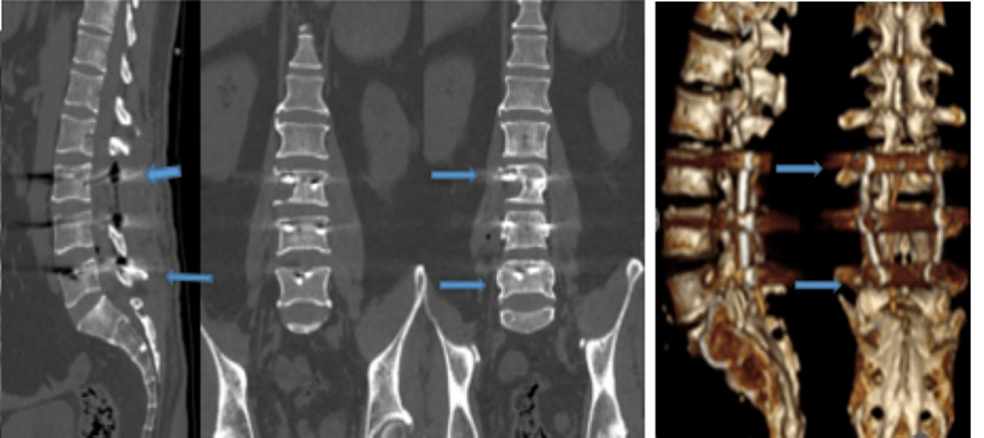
Computed tomography images control postoperative, from left to right: (A) sagittal, (B) coronal, and (C) 3D reconstruction. The images showing the six screws transpedicular fixation in L3-L4-L5 (blue arrow). 3D, three-dimensional
